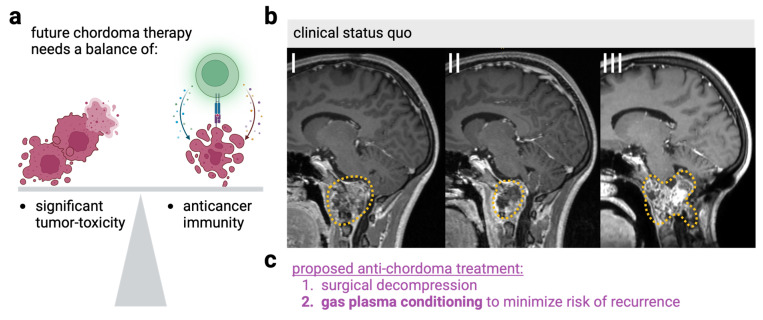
(a) Sketch of the two aspects of direct tumor toxicity and anticancer immunity that are needed to balance future effective chordoma therapies. (b) MRI images (T1-weighted with contrast medium) show the typical clinical course of an aggressive chordoma (I) at time of diagnosis, (II) after surgical decompression of the brain stem and important neuronal structures, and (III) after its recurrence as extensive disease. (c) Summary of the proposed chordoma treatment that adds gas plasma oxidation after surgical decompression to minimize the risk of tumor recurrence. Figure copyright is by the authors.
